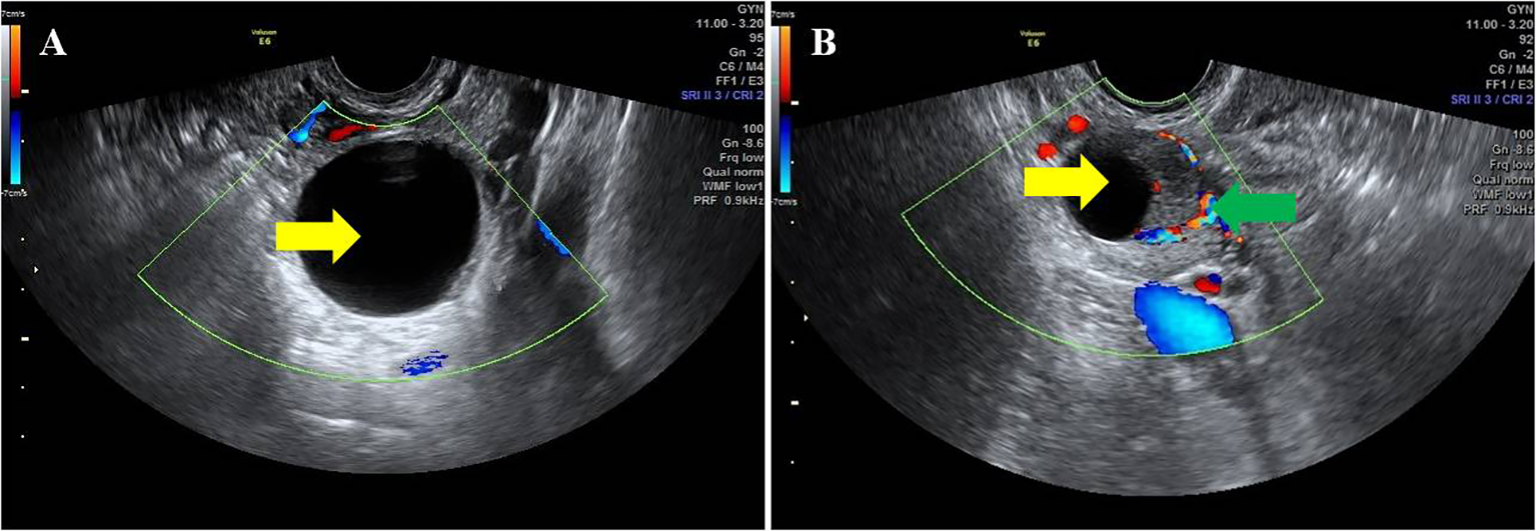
Ultrasonic characteristics of different ovarian cysts. The yellow arrow indicates ovarian cysts. The green arrow indicates the hemicycle blood flow around the corpus luteum. (A) Follicular cyst. (B) Corpus luteal cyst.
